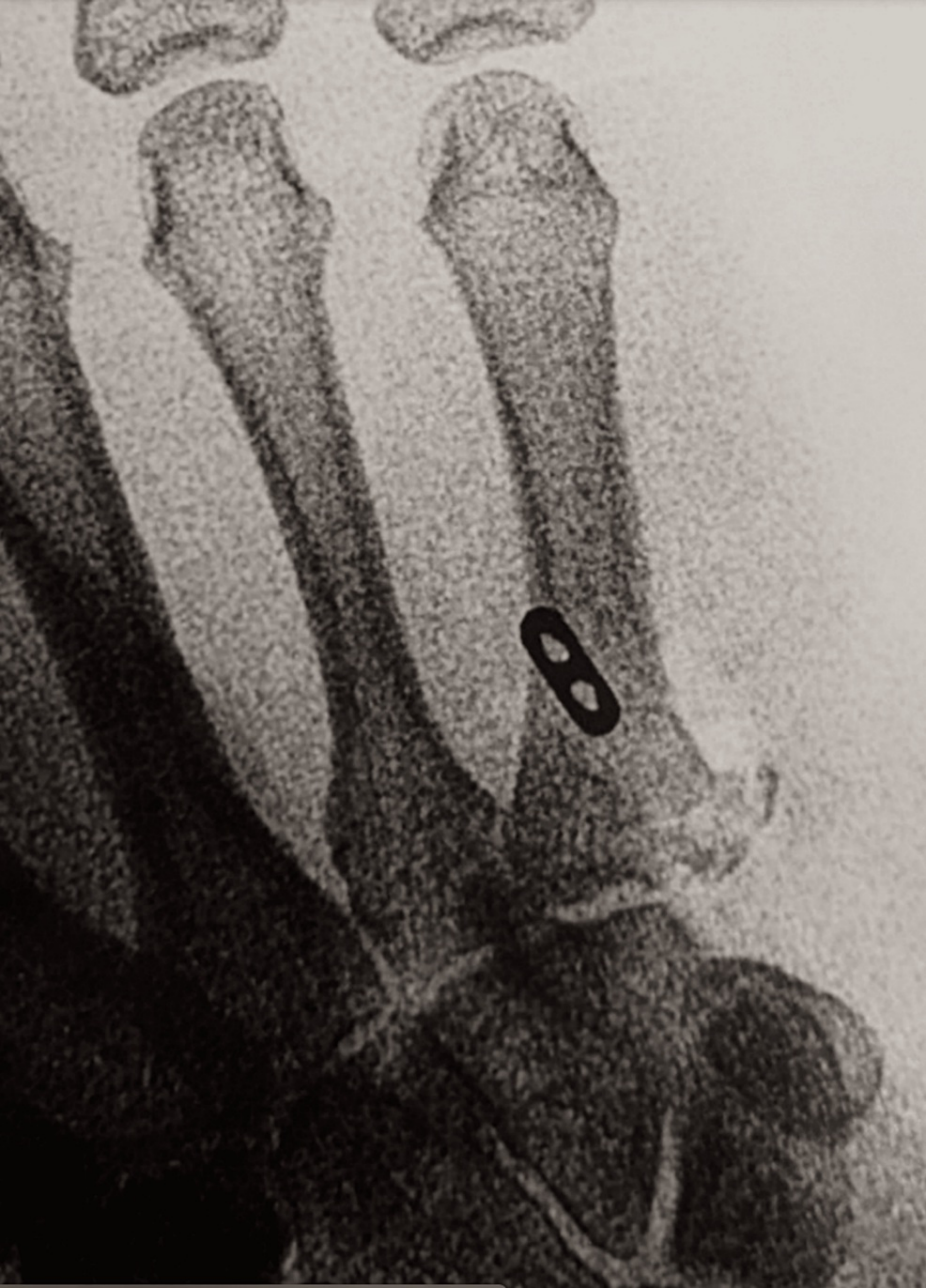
Intra-operative PA radiographs of the ECU repair with suture button. ECU: extensor carpi ulnaris
